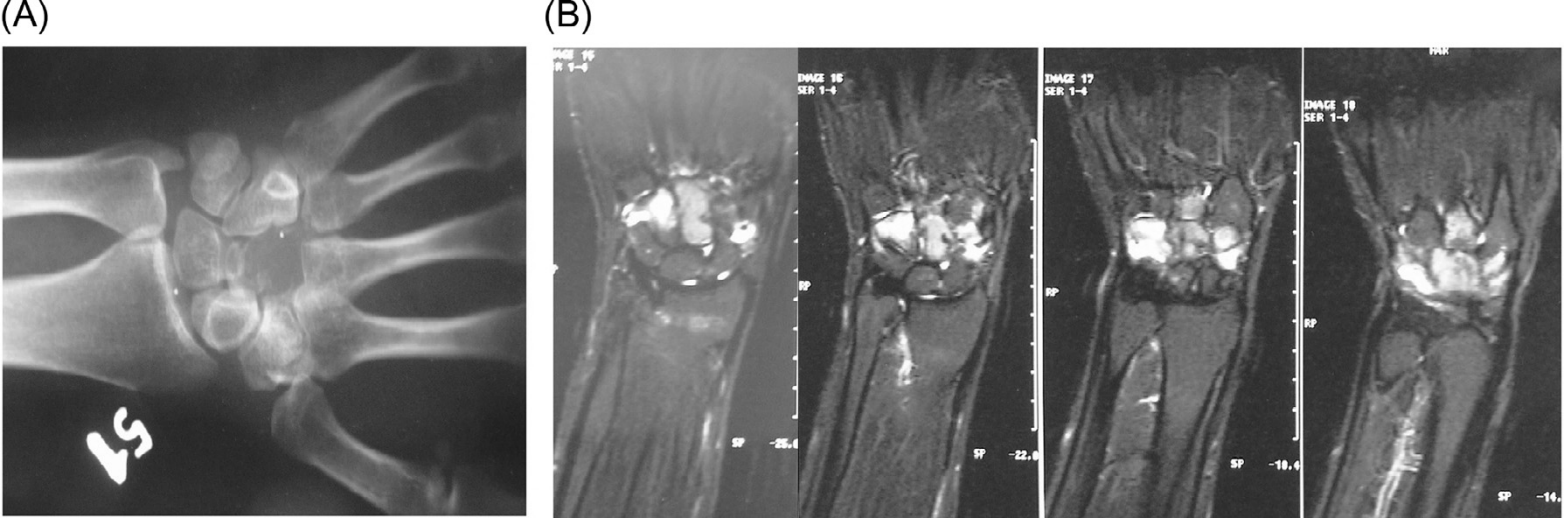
(A) Preoperative roentgenogram of the left wrist, (B) preoperative MRI of the wrist.
